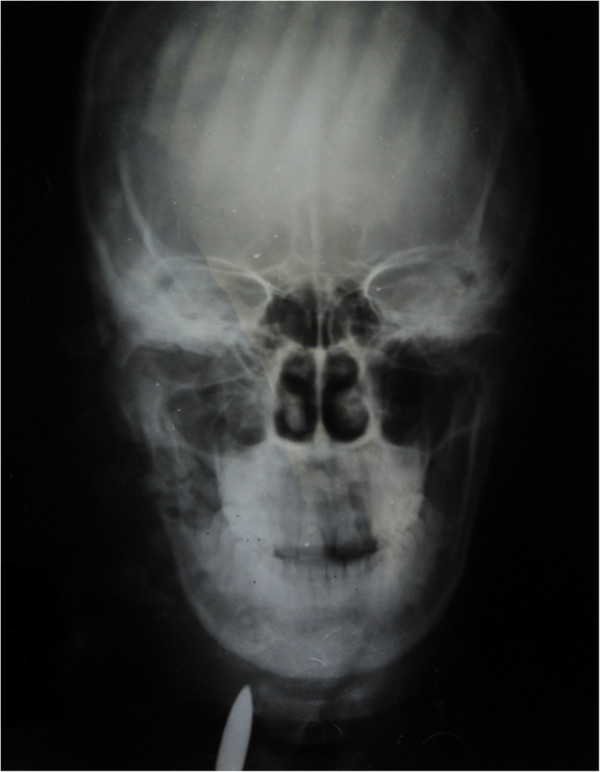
Anteroposterior radiograph of the head and neck. This shows a bullet in the right side of the neck. Comminuted fracture of the mandible (angle and ramus) and maxillary antrum are revealed.
